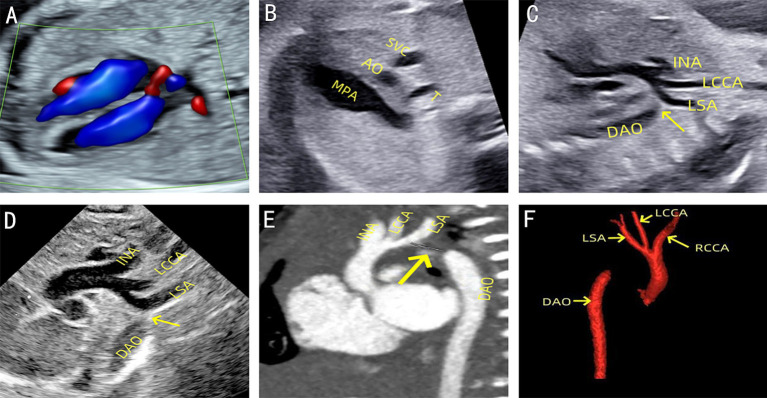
IAA Type A Prenatal Ultrasound and Postnatal Imaging. (A) The left and right heart proportions are basically symmetrical. (B) The pulmonary artery is significantly larger than the aorta, and the internal diameter of the aorta is slightly smaller than that of the superior vena cava. (C) The aortic arch gives off the left subclavian artery and then breaks continuity with the descending aorta. (D) Postpartum echocardiogram, arrow indicates interruption location. (E) Postpartum CTA imaging, with arrows indicating the location of the interruption. (F) Postpartum CTA three-dimensional reconstruction.
